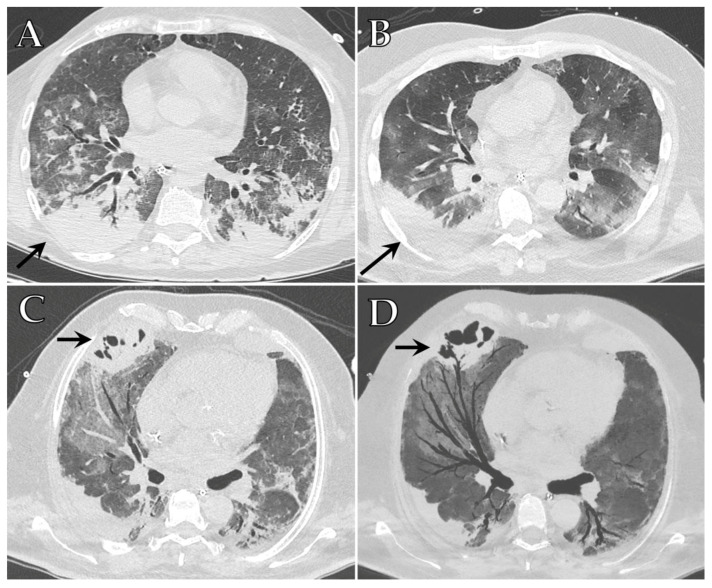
Axial HRCT images of different patients admitted to ICU demonstrating a sudden increase of large consolidations (black arrows) (A,B) associated with worsening of COVID-19 pneumonia. Superimposed infections of *Acinetobacter* (A) and *Aspergillus* (B) were detected. A different COVID-19 patient showed pulmonary consolidation complicated by cavitation located in the medium lobe (black arrow) (C), which demonstrates communication with the bronchial tree at minimum intensity projection (MIP) reconstruction (black arrow) (D); superinfection of *Pseudomonas aeruginosa* was detected.
